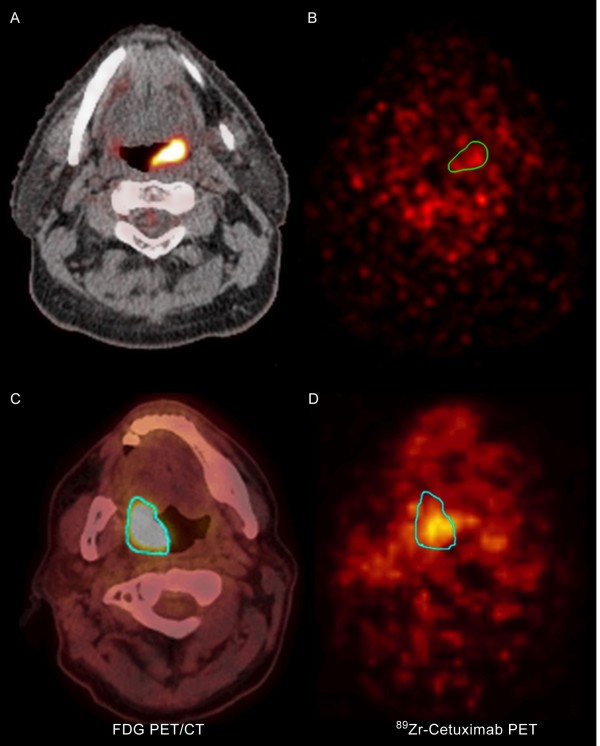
Tumour imaging: FDG-PET/CT scans and ^89^Zr-Cetuximab-PET scans. A + B: patient with a negative ^89^Zr-Cetuximab scan. C + D: patient with a positive ^89^Zr-Cetuximab scan.
